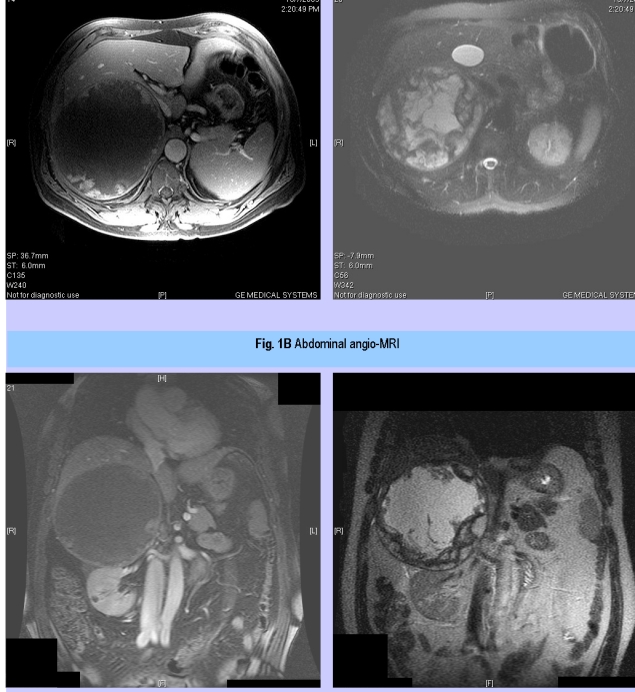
Abdominal angio–MRI: giant adrenal right tumor with liquid areas (A–transversal section, B– axial section)
